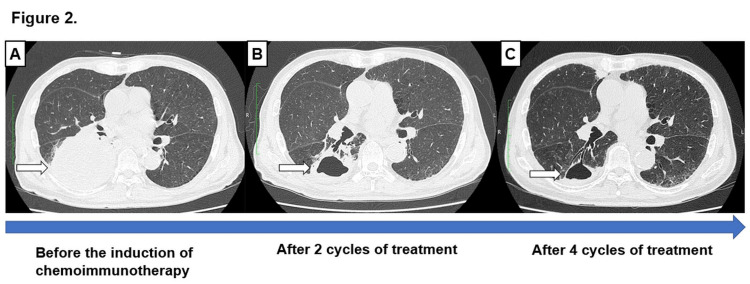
CT axial images (A) CT before the induction of chemoimmunotherapy. (B) CT after two cycles of treatment. (C) CT after four cycles. Remarkable regression of the lower lobe mass lesion of the right lung (arrowhead). CT, computed tomography
